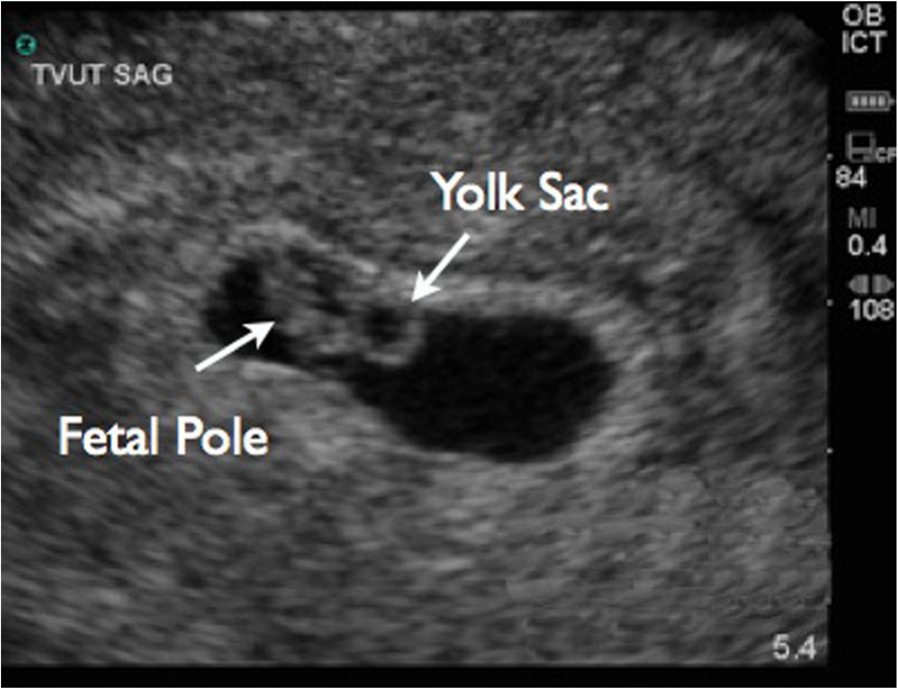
Trans-vaginal ultrasound image showing yolk sac and fetal pole.
